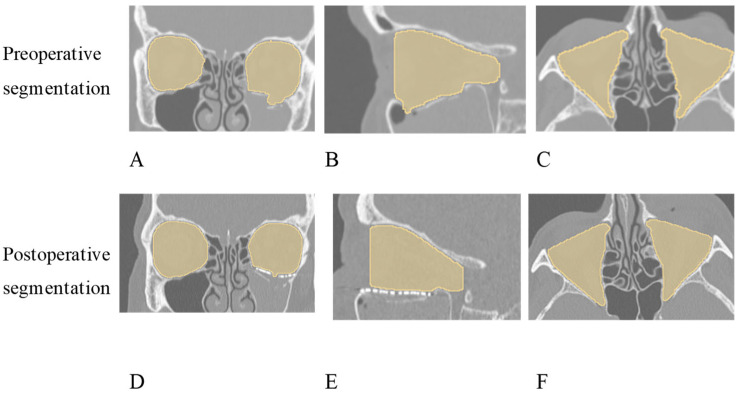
Computed tomography images of a patient treated with a pre-bent titanium mesh. (A)—Pre-operative coronal series, (B)—Pre-operative sagittal series, (C)—Preoperative axial series (D)—Postoperative coronal series, (E)—Postoperative sagittal series, and (F)—Postoperative axial series.
